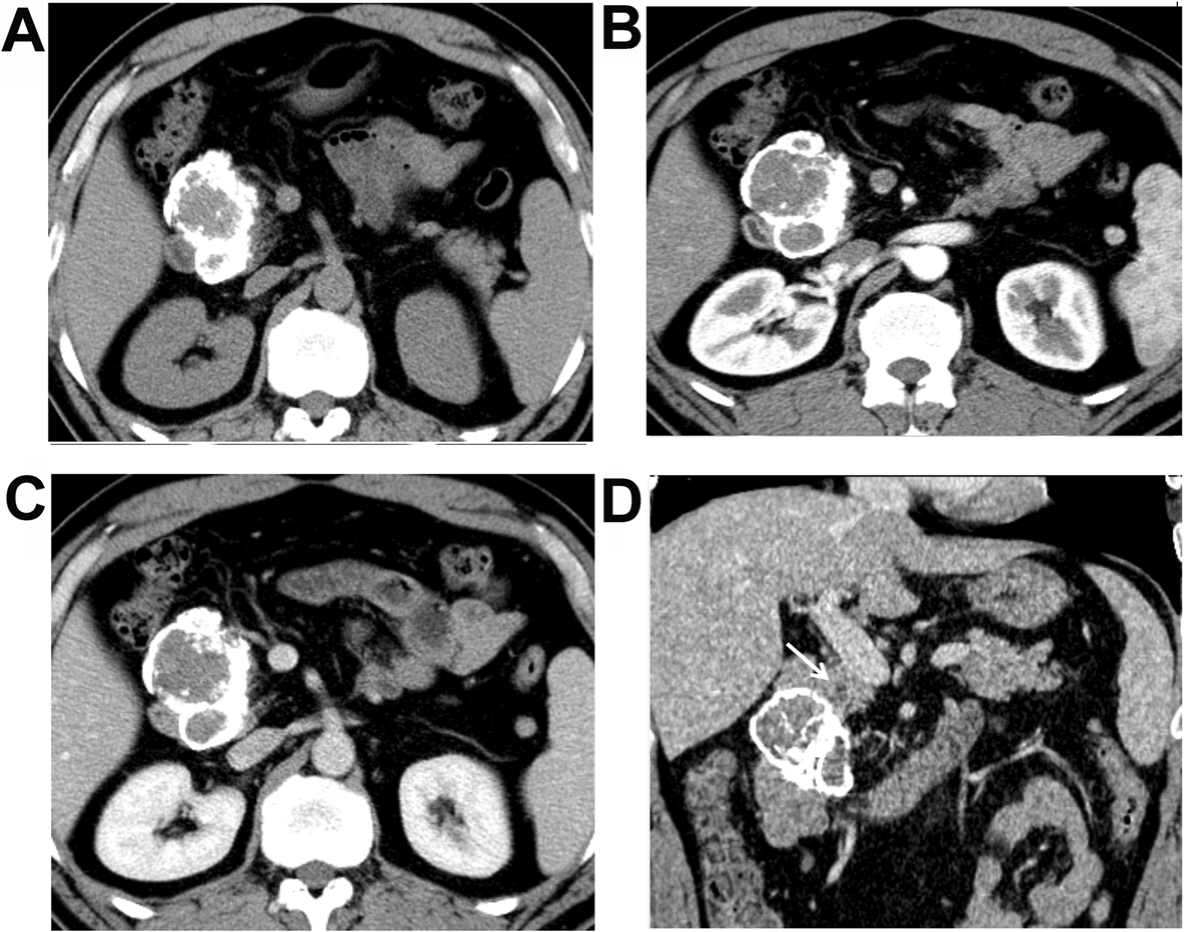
A 49-year-old male patient with discomfort in the upper abdomen. a Plain CT scanning + enhanced CT scanning showed pseudo-papillary neoplasm of pancreas (SPNP). a Plain scanning showed round solid changes at the pancreatic head, of which the boundaries were clear, and eggshell calcification was shown around the mass. b Low enhancement of the lesion in the arterial phase, which was lower than the normal pancreas. c Persistent enhancement of the lesion in the portal venous phase was shown. d Dilation of the pancreatic tube was shown on the reconstructed coronal image (white arrow)
